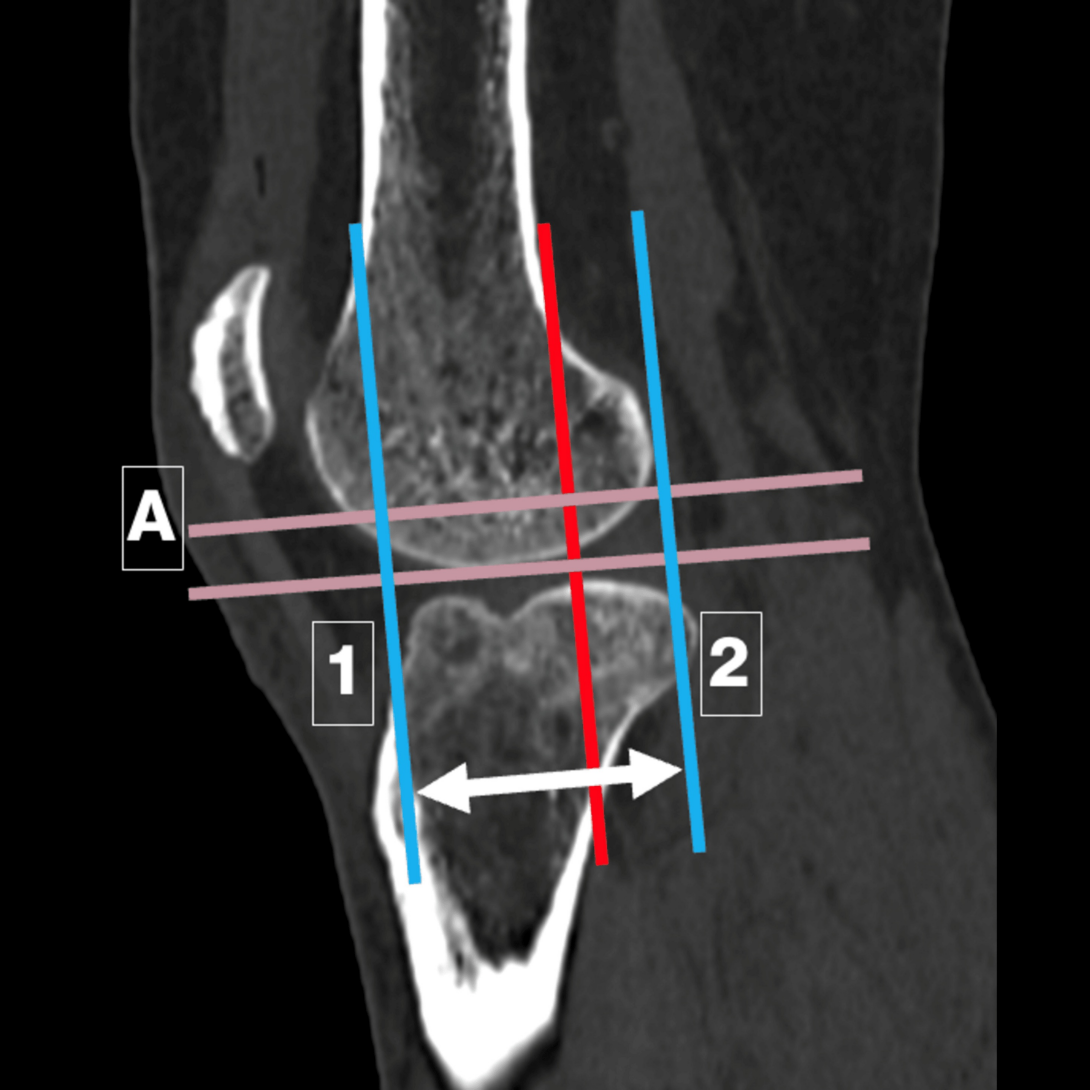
Sagittal CT image to measure the anteroposterior (AP) dimensions and compare them with implant sizes. Red line: drawn across the posterior femoral cortex.
